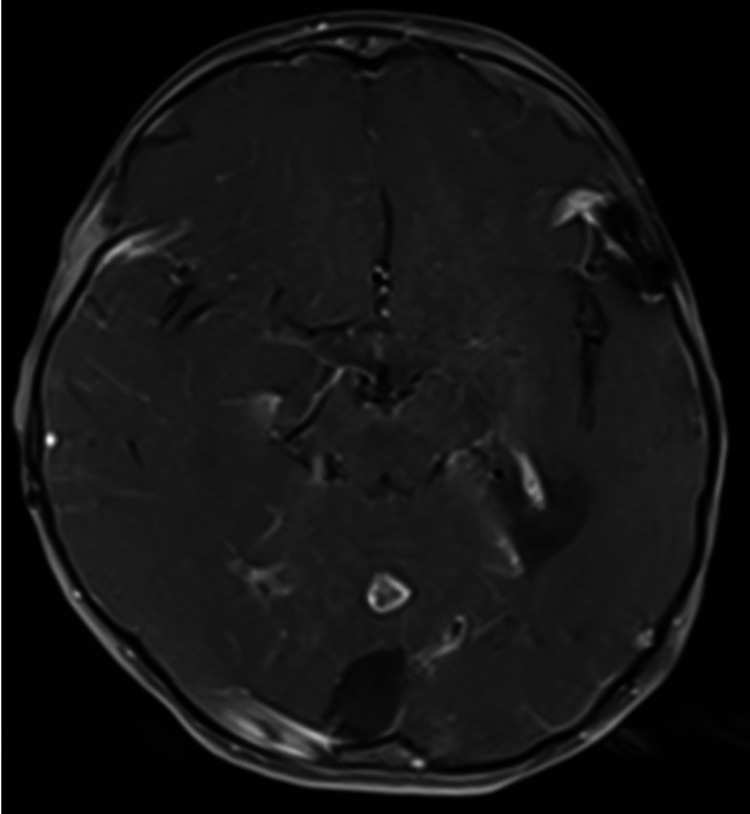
Axial contrast T1 MRI with new enhancement in the previously seen cystic lesion within the cerebellar vermis.
